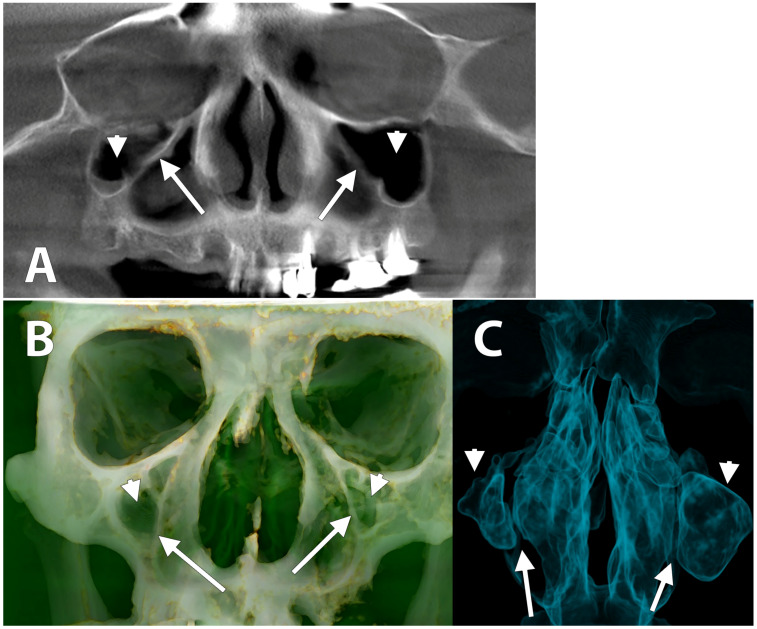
Bilateral maxillary sinus hypoplasia, anterior views. (A) Digital orthopantomogram; (B) three-dimensional volume rendering (Bone+Skin Filter); (C) three-dimensional volume rendering (Airways Filter). Hypoplastic maxillary sinuses (arrowheads) and the lateral nasal walls (arrows) are indicated.
